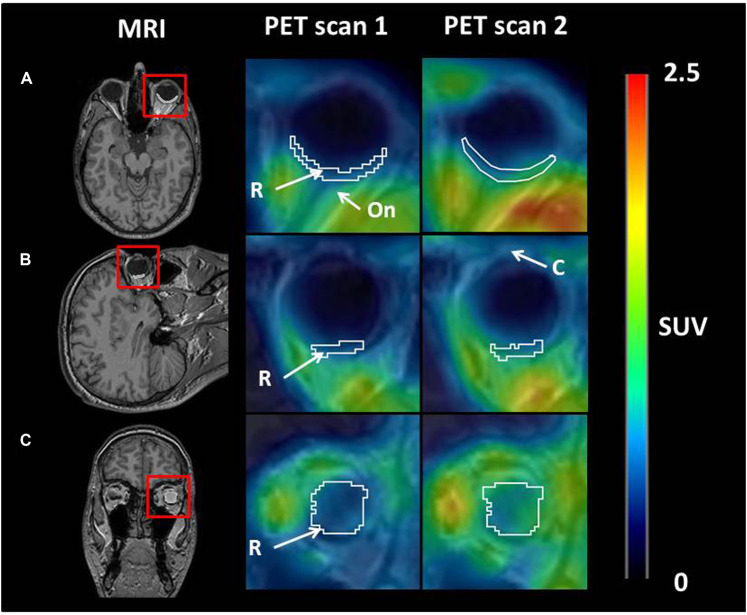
Axial (A), sagittal (B), and coronal (C) planes of representative MR and PET average images (0–60 min) at baseline (scan 1) and during ABCB1 inhibition (scan 2) in one c.421CA carrier. Red rectangles on MR images indicate magnified area on PET images. A representative region of interest for retina (white contour) is shown. Anatomical structures are labelled with arrows: R, retina; On, optical nerve; C, cornea. Radiation scale is expressed as standardized uptake value (SUV) and set from 0 to 2.5.
